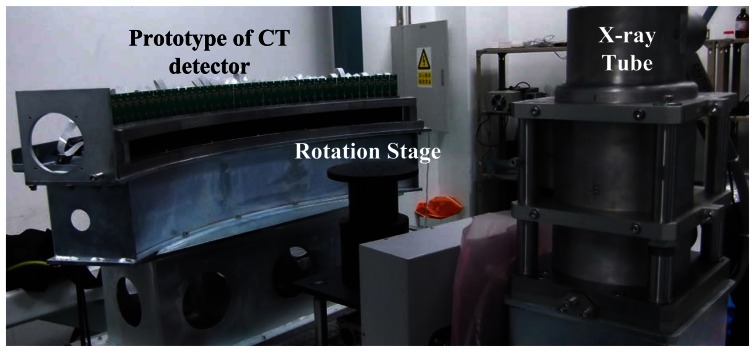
Setup of the CT detector tests.
